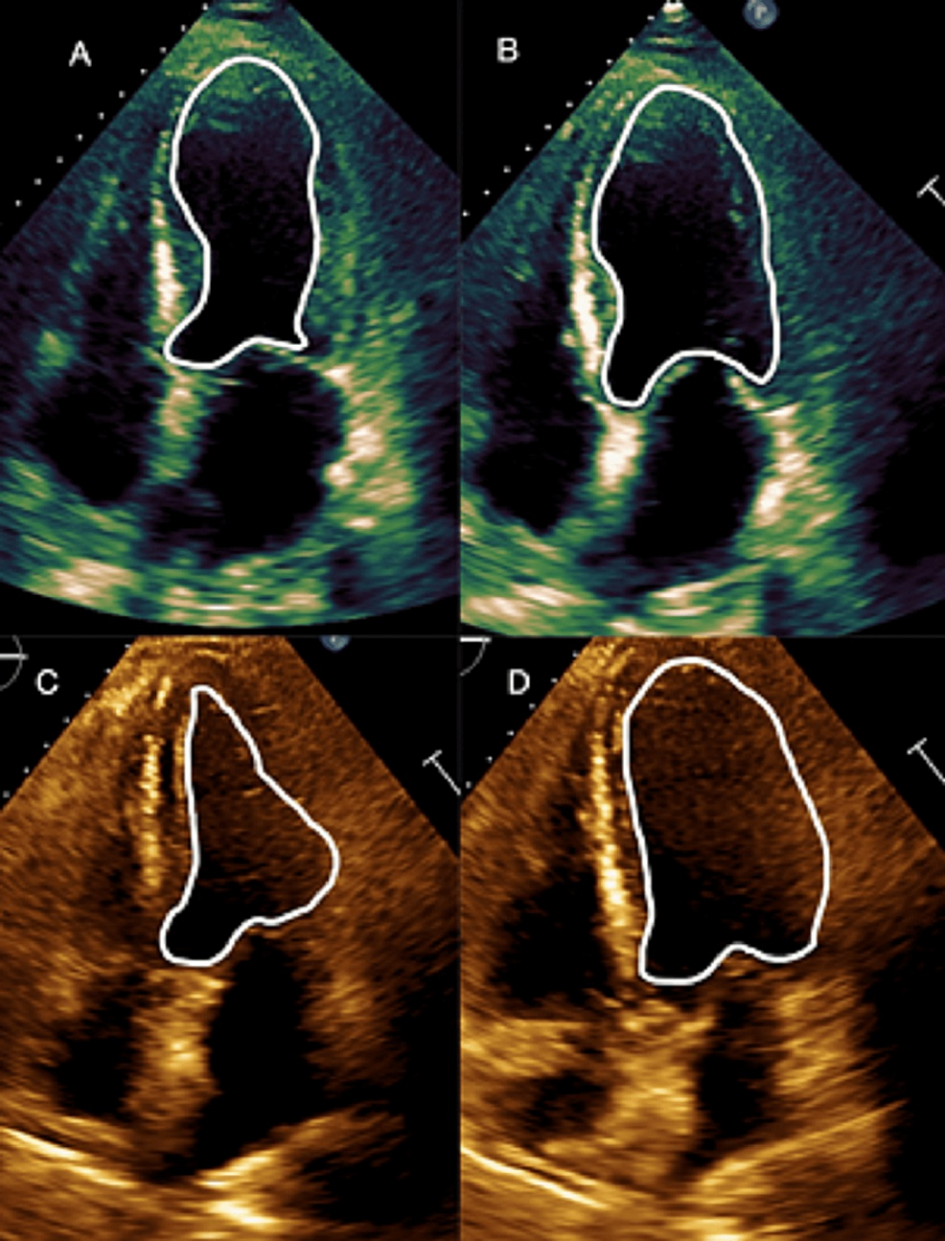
Echocardiography findings depicting the left ventricle A: Pre-treatment systole displaying apical and mid-ventricular hypokinesis, B: Pre-treatment diastole, C: Post-treatment systole displaying appropriate left ventricle (LV) contraction, D: Post-treatment diastole
